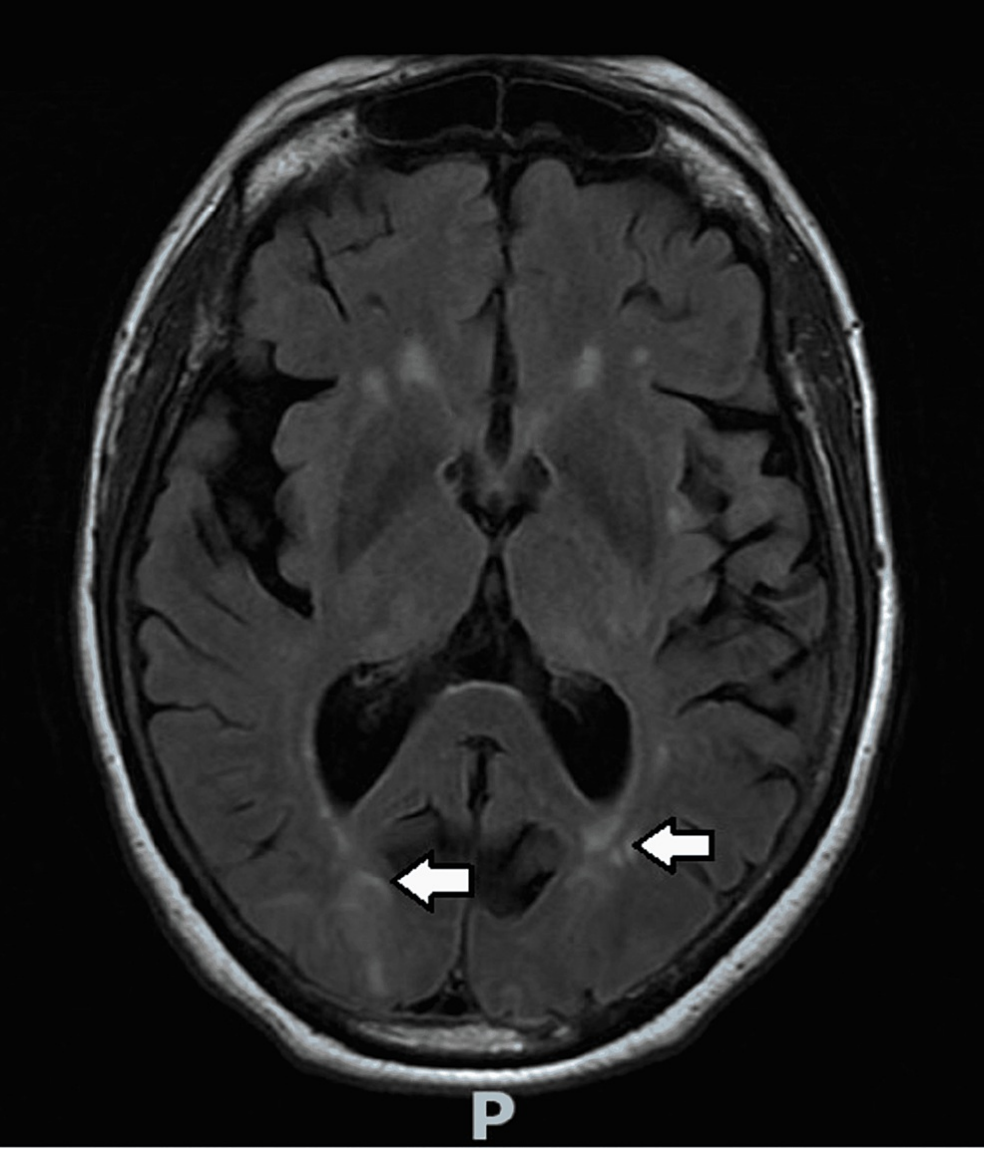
MRI of the brain without contrast The white arrows represent interval development of T2/fluid-attenuated inversion recovery (FLAIR) hyperintensities in the parieto-occipital lobes, which represent acute posterior reversible encephalopathy.
